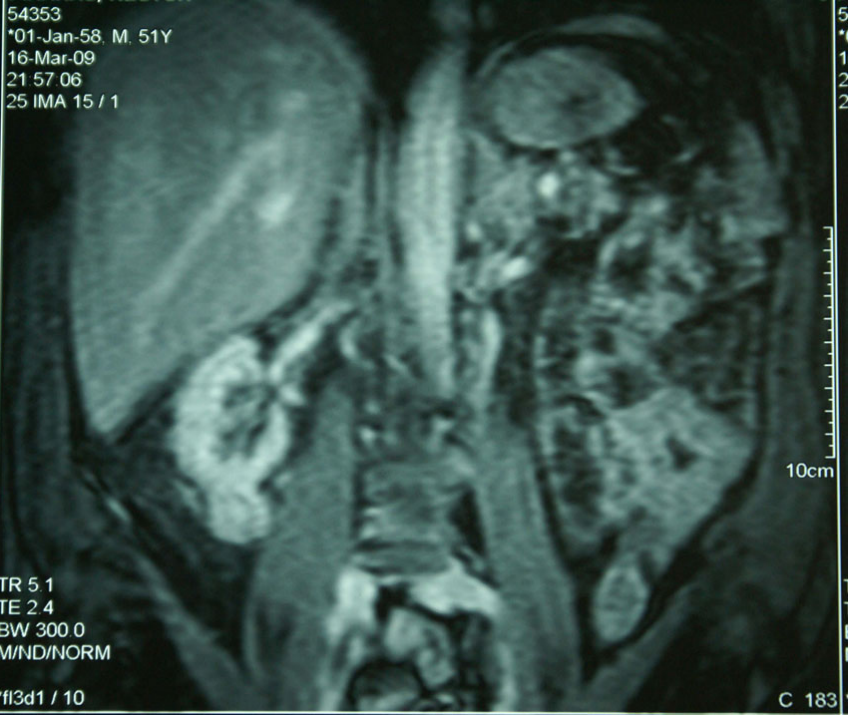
Magnetic resonance imaging post contrast (MRI) scan also showing a 2,5 × 2,7 cm mass at the lower pole of the right kidney and an adenoma of the right adrenal gland.
